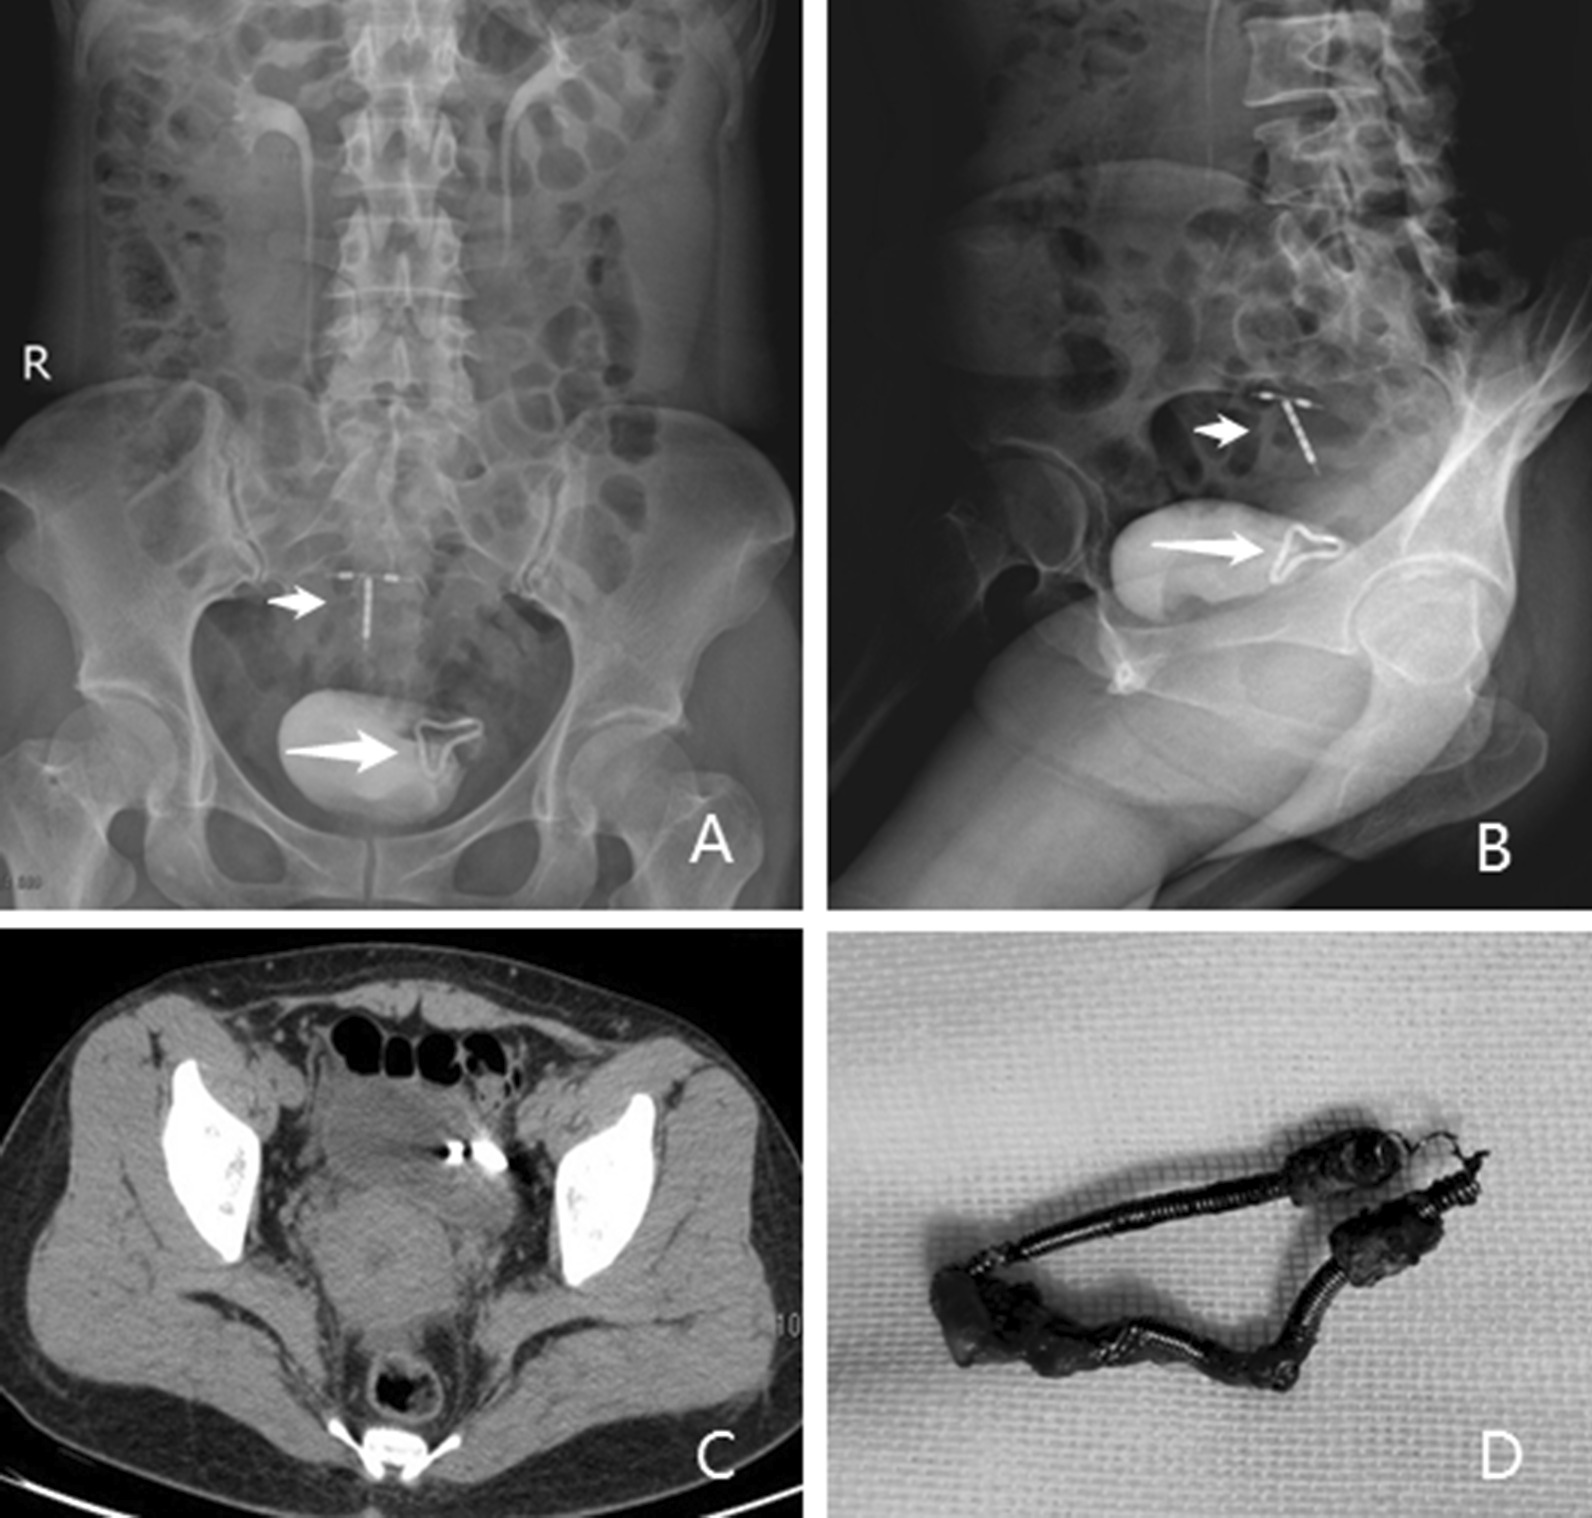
IUDs of case NO.2 (a and b: IVP demonstrating two IUDs in the pelvic cavity, one of them was in the bladder. c: Her CT scan showing an IUD penetrated the left side of the bladder. d: The removed IUD.)
